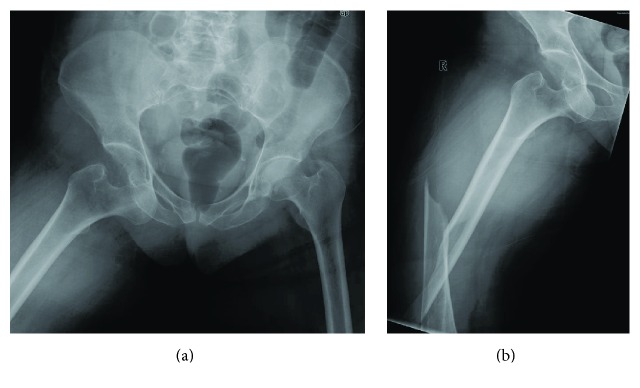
Preoperative X-ray examination.
